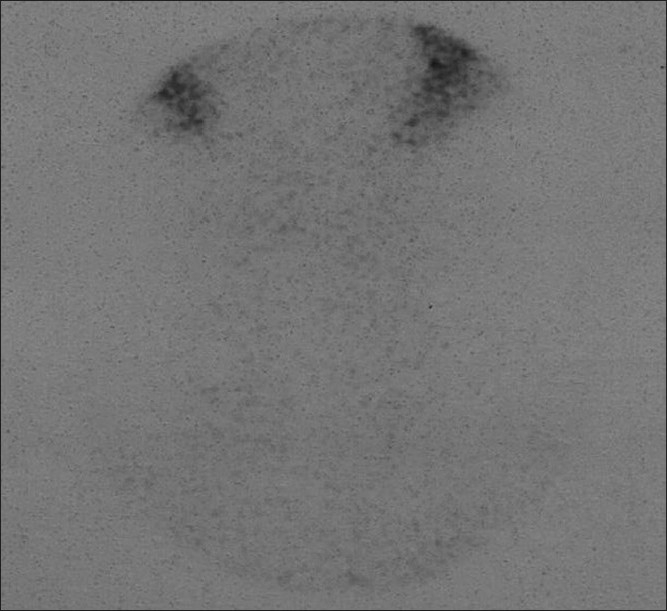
Tc-99m thyroid scintigraphy (after the completion thyroidectomy). No residual thyroid tissue was detected
